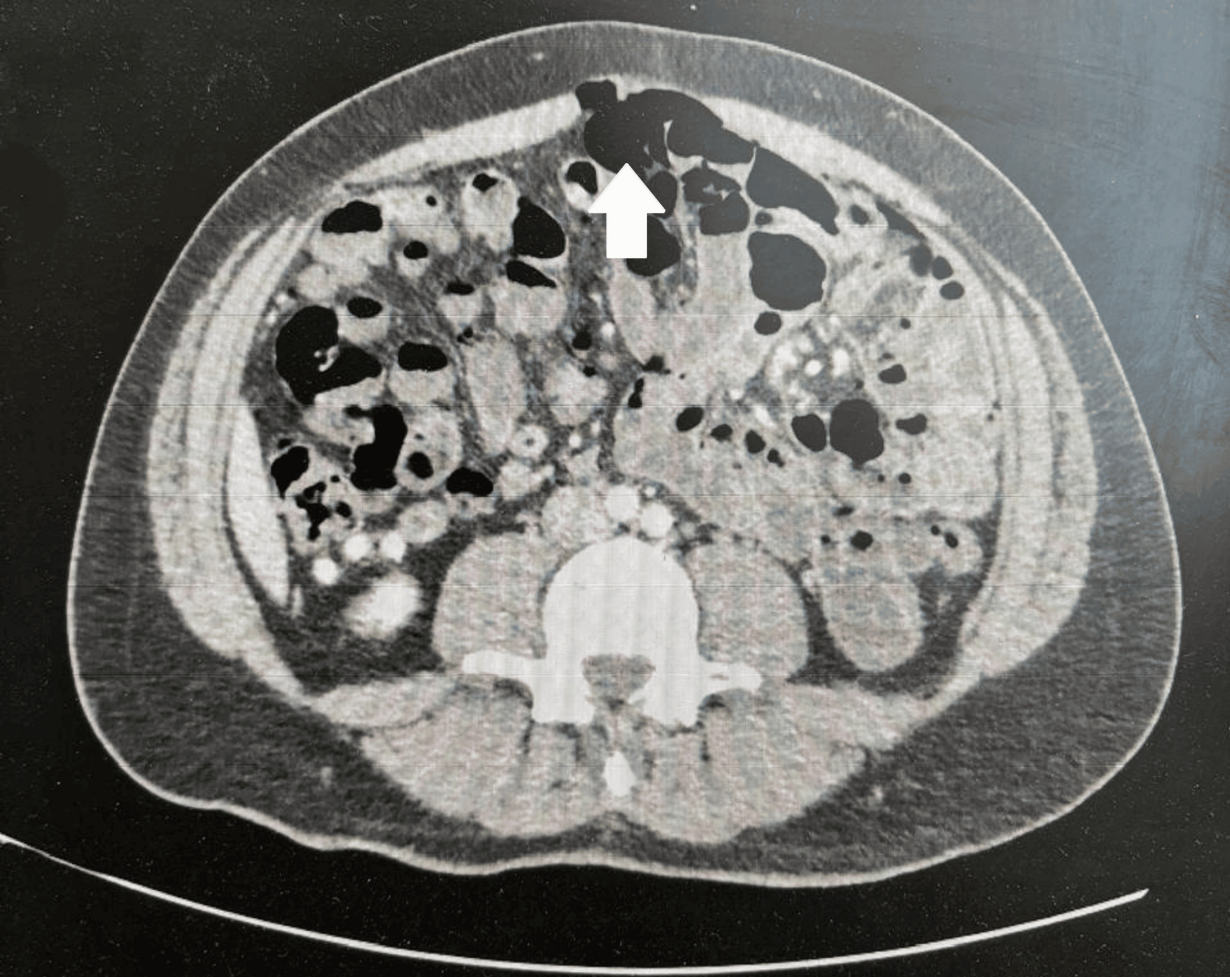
Transverse abdominal CT scan with IV contrast showed pneumoperitoneum due to perforated jejunal diverticula.
